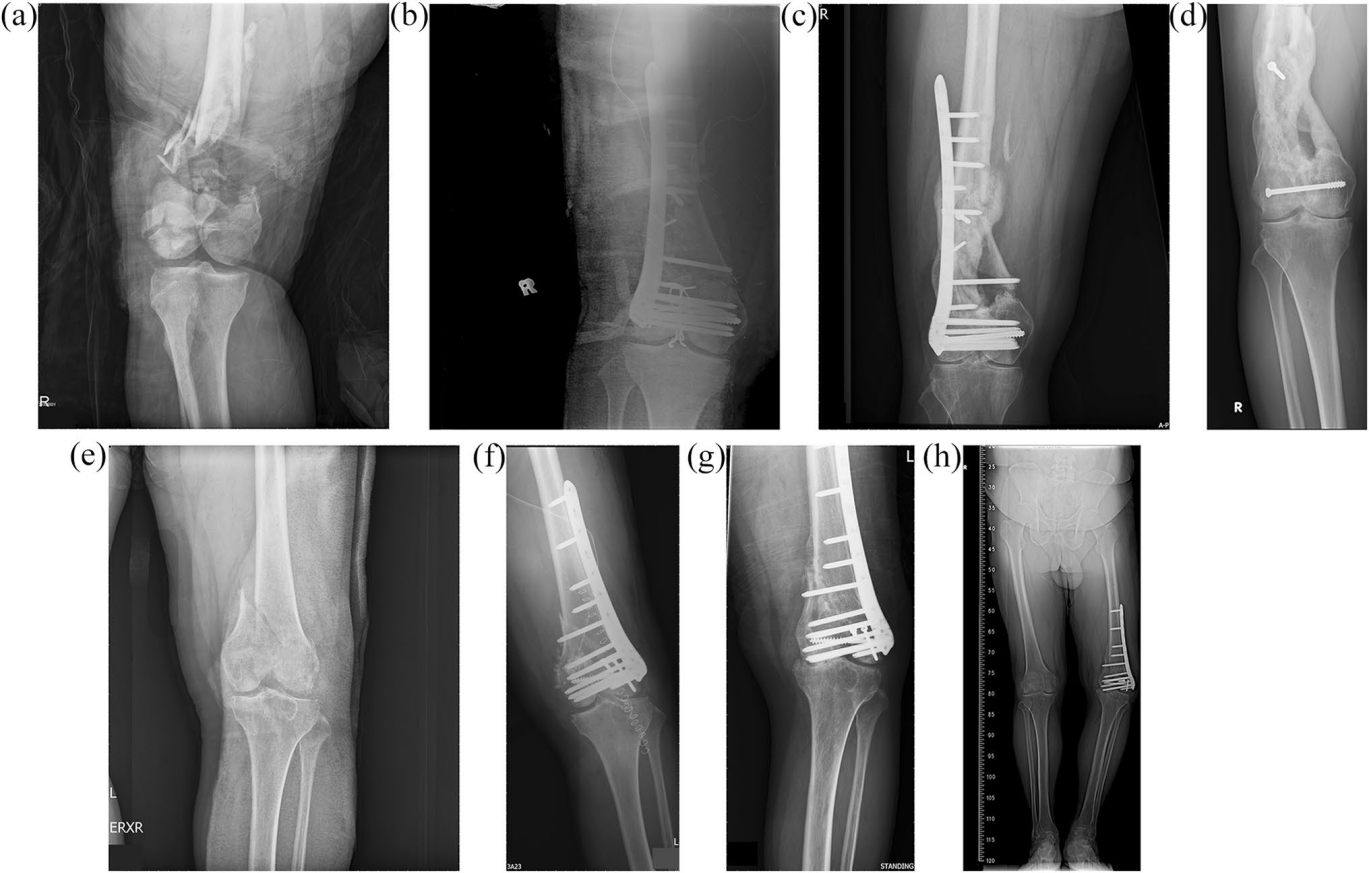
The demonstration of varus collapse with malunion after osteosynthesis with lateral locking plate. (a and b) Pre and post-operative anteroposterior plain film of an AO type C3, open type IIIa distal femoral and patellar fracture in a 27-year-old male. c Varus collapse with loosed screws over the femoral shaft at 27 months after the operation. d Malunion with varus deformity at 90 months. The change of LDFA was 11˚. e and f Pre and post-operative anteroposterior plain film of a closed AO type C3 left distal femoral fracture in a 50-year-old male. g and h Varus collapse of the medial femoral condyle with malunion at 14 months after the operation. The change of LDFA was 5˚
